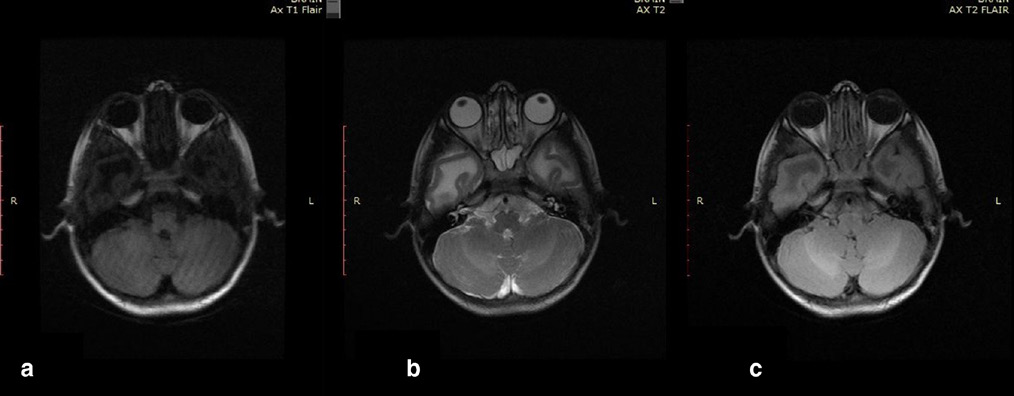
Axial *T*_1_WI (Figure 4a), Axial *T*_2_WI (Figure 4b) and axial FLAIR image (Figure 4c) of brain of the first child showing sparing
